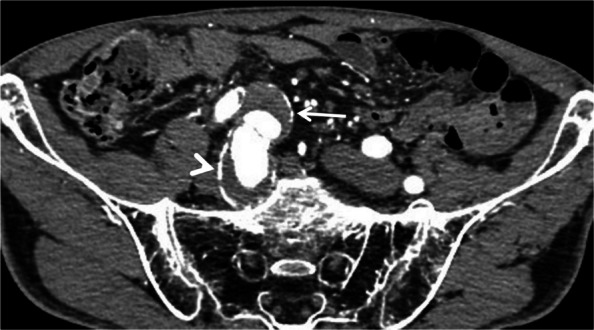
Axial preoperative contrast-enhanced CT. Right common iliac artery aneurysm (arrow) and internal iliac artery aneurysm (arrowhead) are observed
